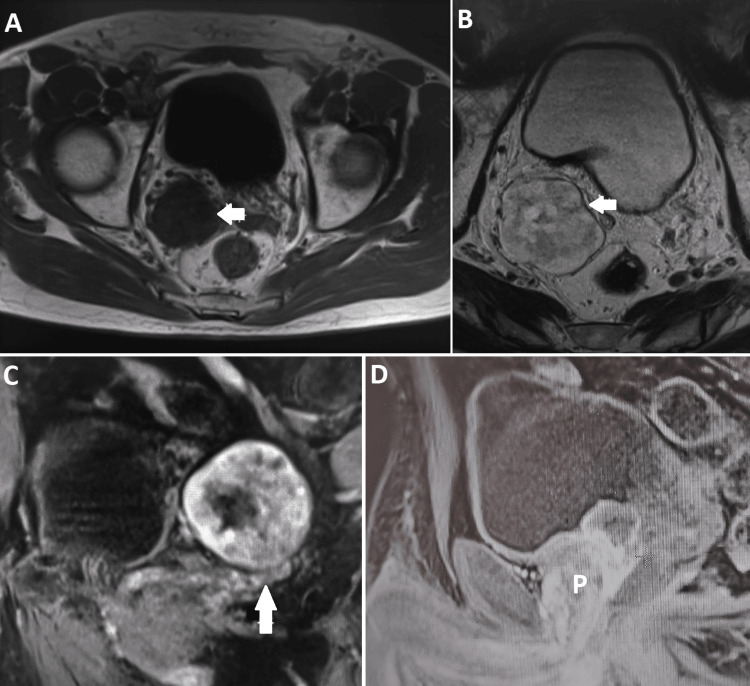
MRI showing nodular and polylobulated mass postero-lateral to the bladder (arrows), in close contact with the right adrenal vesicle. A: T1-weighted MRI showed hyposignal with avid contrast uptake, a small non-enhancing central zone, and restriction to water diffusion in several zones. B: T2-weighted MRI presented heterogeneous hypersignal. C: Sagittal plane. D: Saggital plane: relation with prostate (P).
